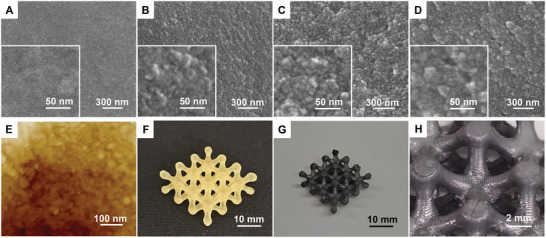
Ceramic PIMS materials. A–D) SEM images (cross‐section) of the pyrolyzed preceramic object (800 °C, under argon) prepared using PLAc‐CTA with *X*
